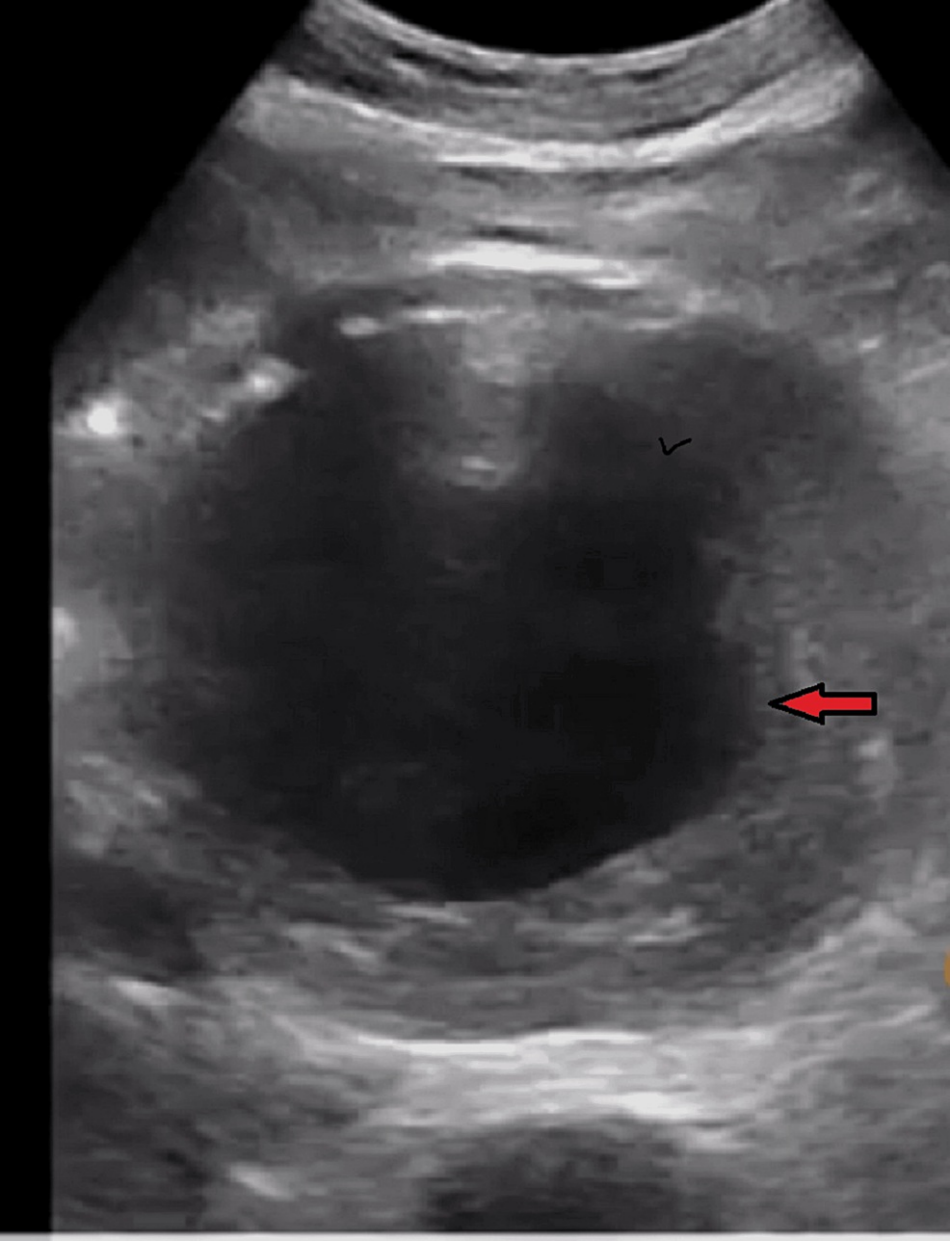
Ultrasound image showing AAA with a lesion measuring 9 cm with circumferential parietal thrombus AAA: abdominal aortic aneurysm
